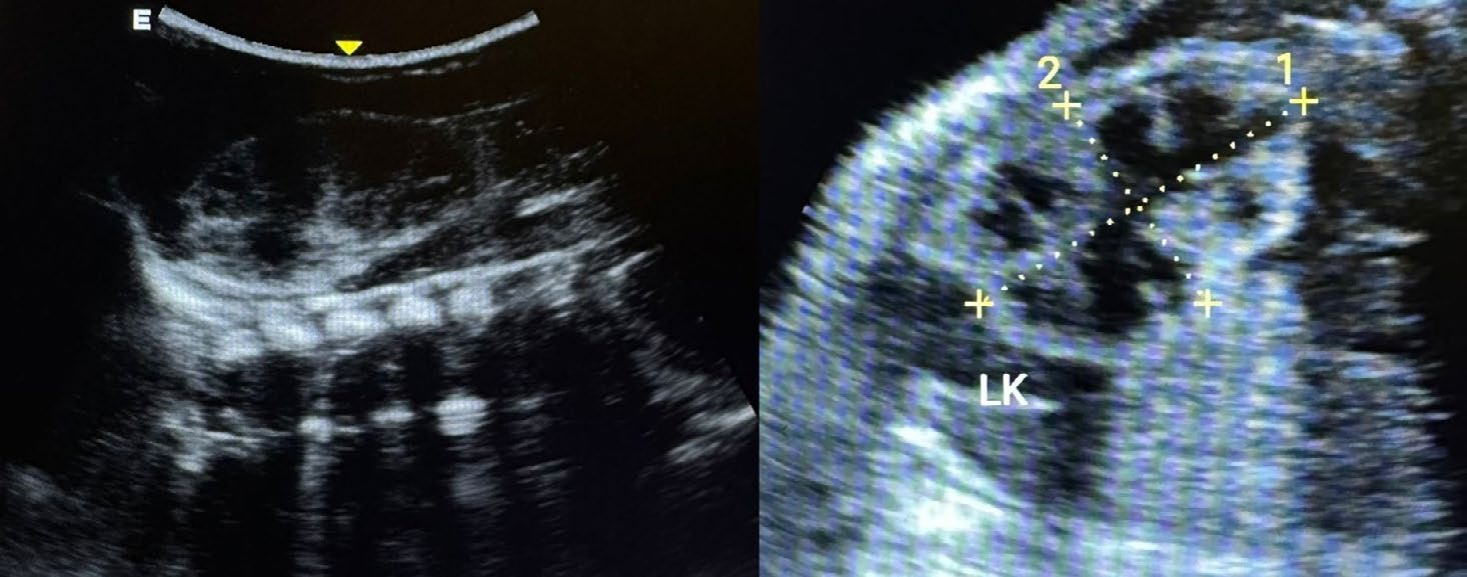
In utero ultrasonography showing bilateral MCDK.
